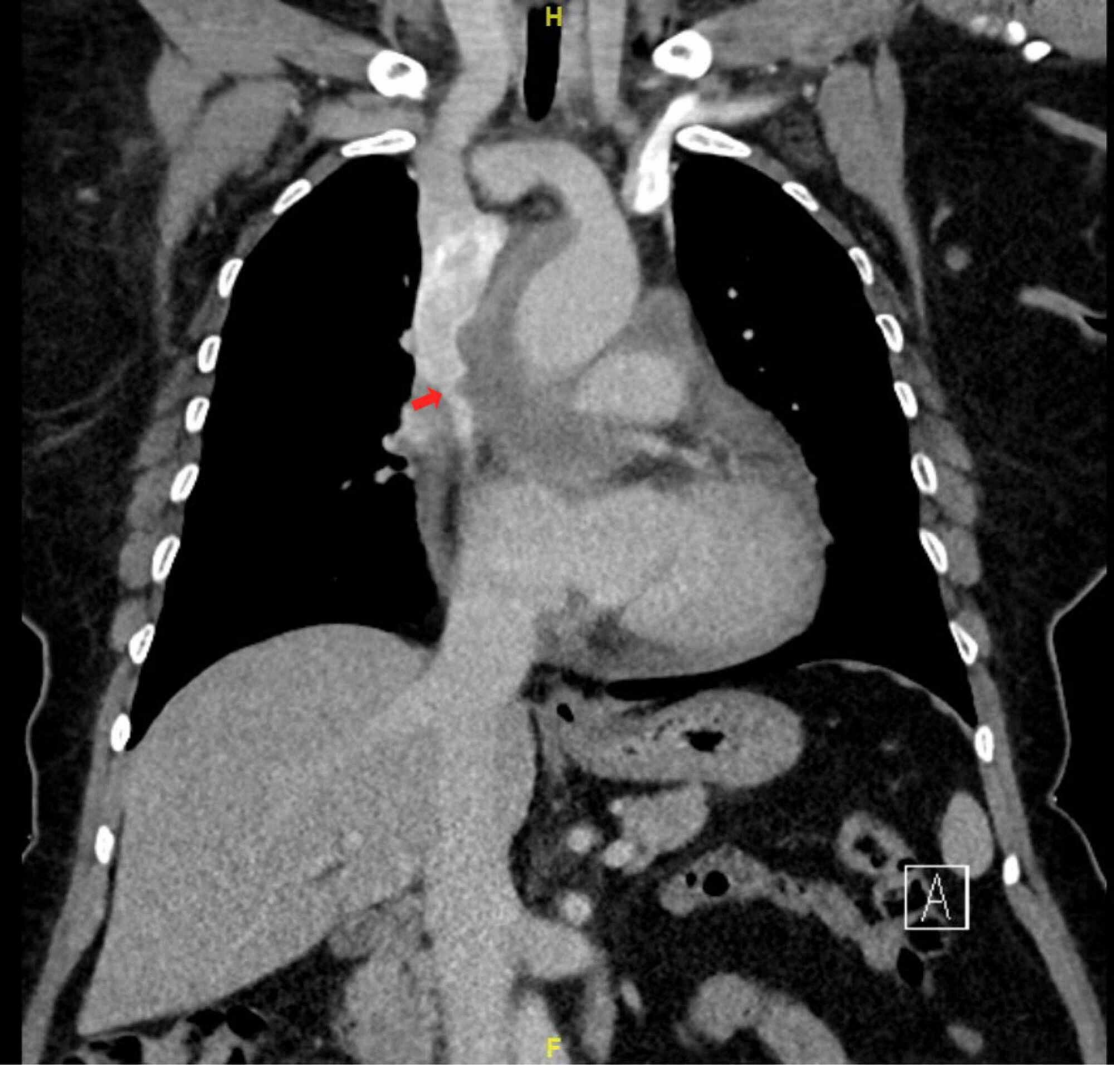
CT chest coronal view: red arrow showing SVC narrowing, pericardial effusion is also noted here. CT: computed tomography; SVC: superior vena cava.
